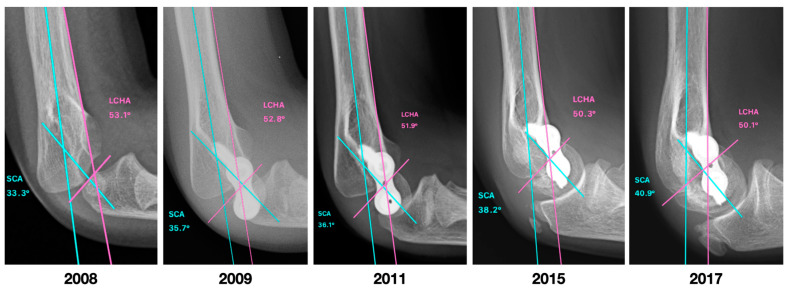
Lateral radiographs of the elbow of the same patient in Figure 5, showing an improvement in the radiological parameters, with values closer to normal in the last radiological control. SCA, shaft-condylar angle, LCHA, lateral capitellohumeral angle.
